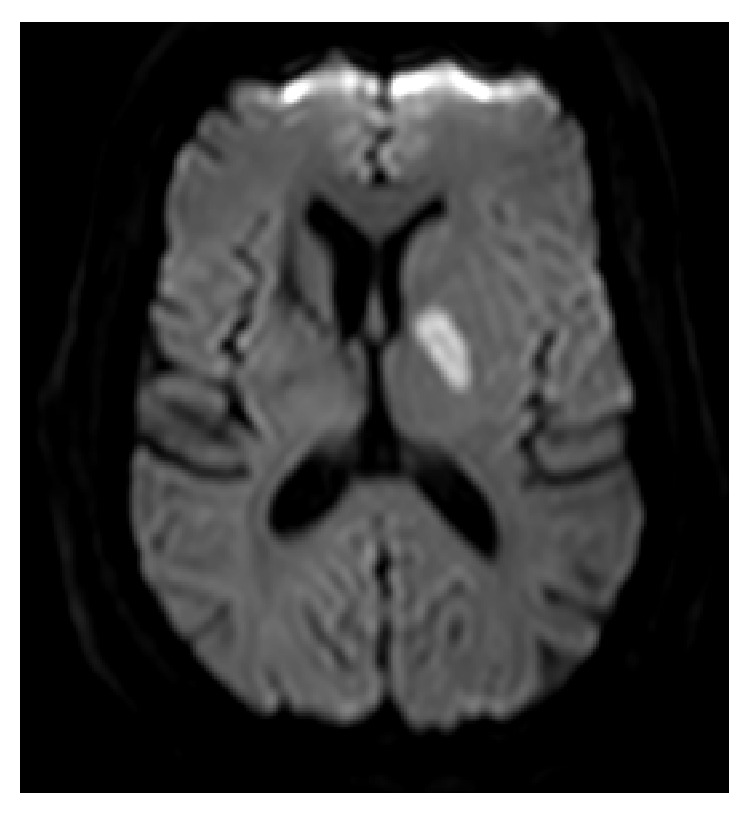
Axial MRI of the brain. Diffusion weighted imaging sequence showing acute left globus pallidus infarct.
